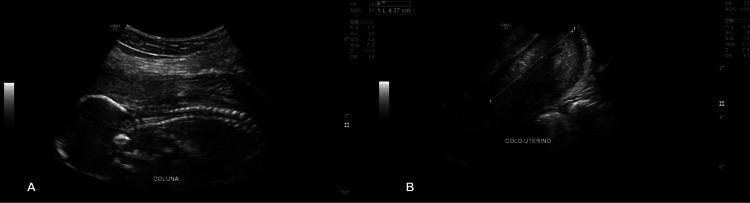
Obstetric transvaginal ultrasound at approximately 19 weeks of gestation showing absolute oligohydramnios (A) and a cervical length of 4.3 cm with a slightly opened internal os (B). FR, frame rate; AO, acoustic output; CHI, coded harmonic imaging; Frq, transducer frequency; Gn, overall gain; S/A, image filtering setting specific to the ultrasound system; D, image depth; DR, dynamic range; os, cervical os.
